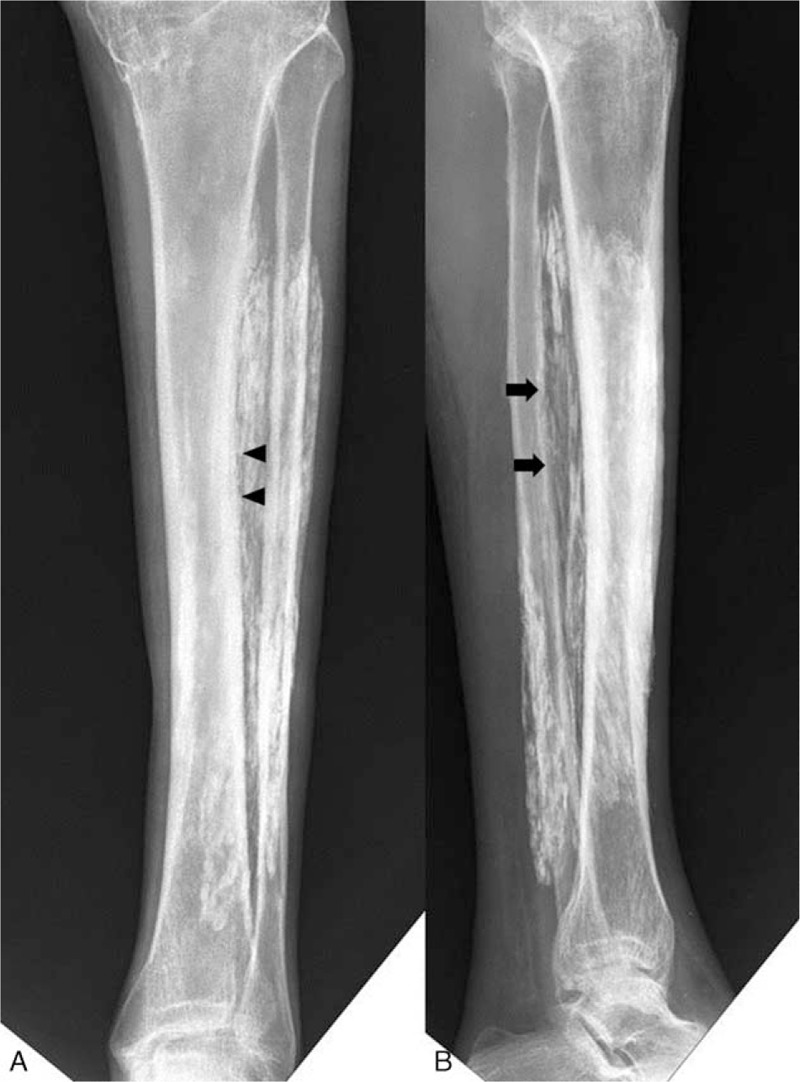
At 18 years after the initial trauma, radiographs showing an extensive radiopaque mass with plaque-like and amorphous calcifications. Anteroposterior view (A) showing sclerotic change with erosion in the lateral cortex of the middle third of the tibia (arrow head). Also lateral view (B) showing sclerotic with erosion in the anterior cortex of the middle third of the fibula (arrow).
